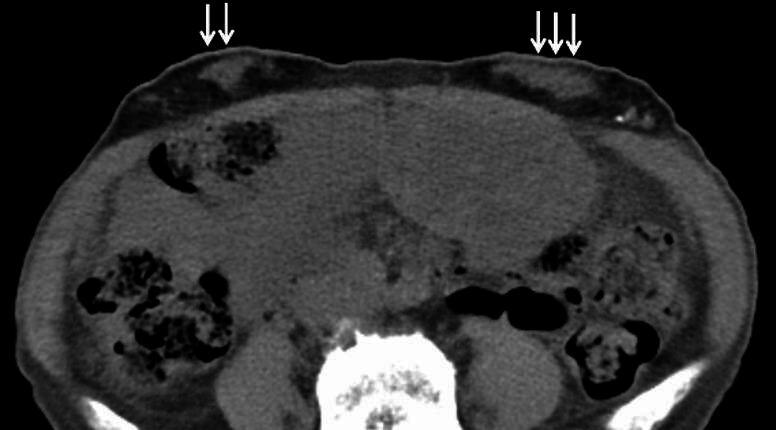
Insulin ball. A man in his 80s with asymptomatic type 2 DM had been subcutaneously injected with insulin for a long time. CT shows heterogeneous subcutaneous nodules (arrow). Abbreviations: CT, computed tomography; DM, diabetes mellitus
